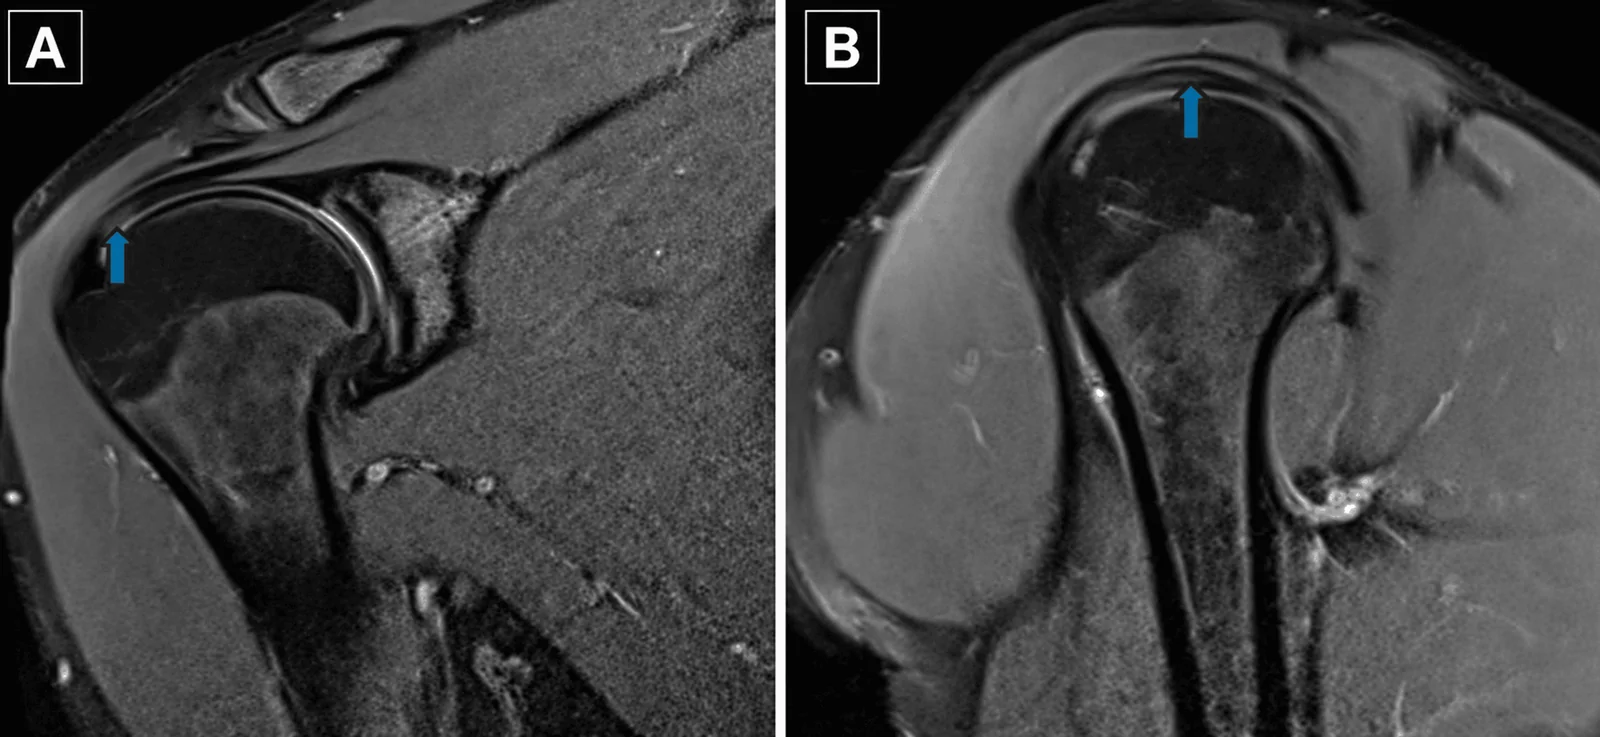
Supraspinatus tendinosis on coronal and sagittal MRI A: coronal proton density fat-saturated MRI image demonstrates a patchy intratendinous hyperintense signal within the supraspinatus tendon without definite extension to the articular or bursal surface (arrow); B: sagittal proton density fat-saturated MRI image shows preserved tendon fiber continuity and alignment, consistent with supraspinatus tendinosis (arrow).
